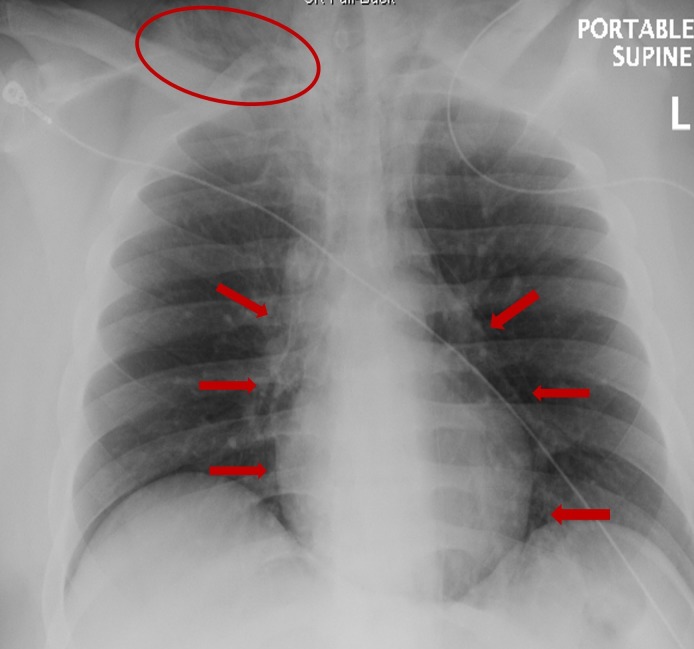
Chest x-rays: pneumomediastinum and subcutaneous emphysema at the right base of the neck Circle: emphysema; Arrows: pneumomediastinum.
